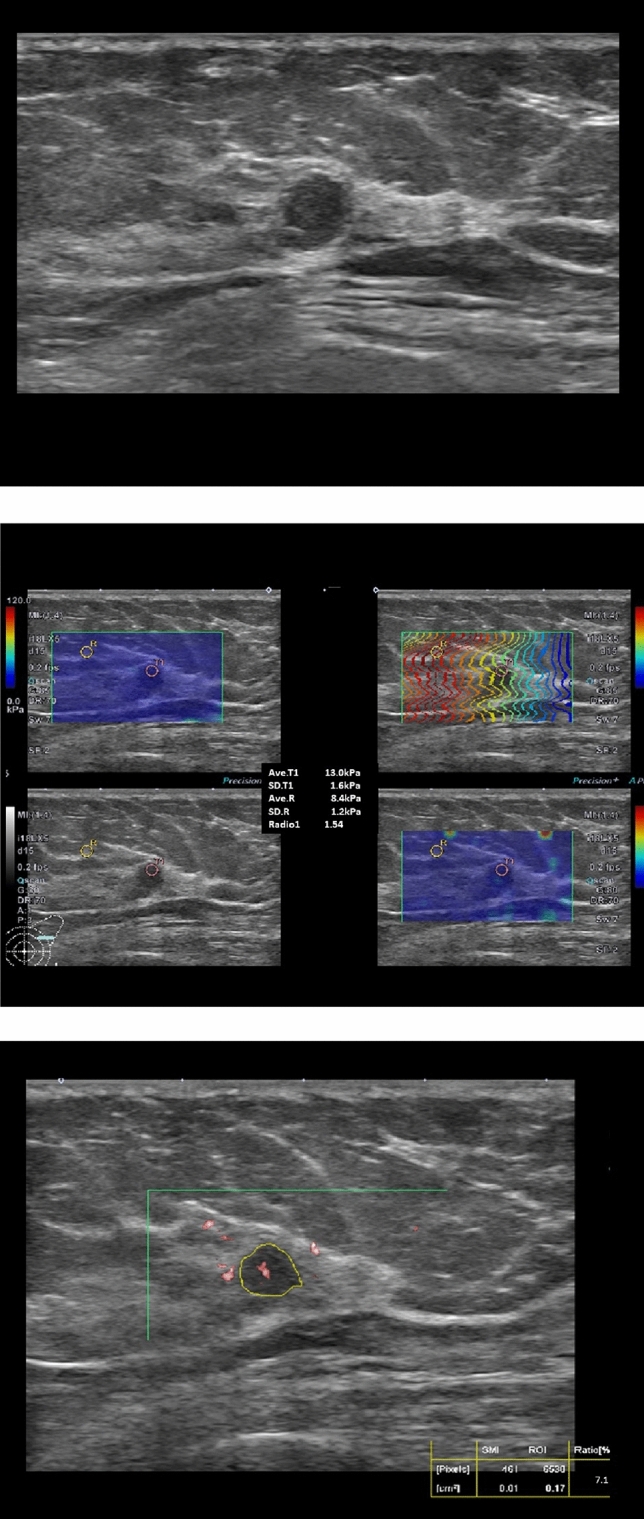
DCIS in a 50-year-old female patient. (A) B-mode ultrasound showed a 0.6 m-sized, mass categorized as C4a. (B) The lesion had soft elasticity measured below the cutoff values as SWEmax 13.0 kPa and SWEratio 1.54. (C) The SMI vascular index was measured at 7.1%, over the cutoff value. The combined score was 3, representing a benign lesion, but the pathologic diagnosis was DCIS, intermediate.
